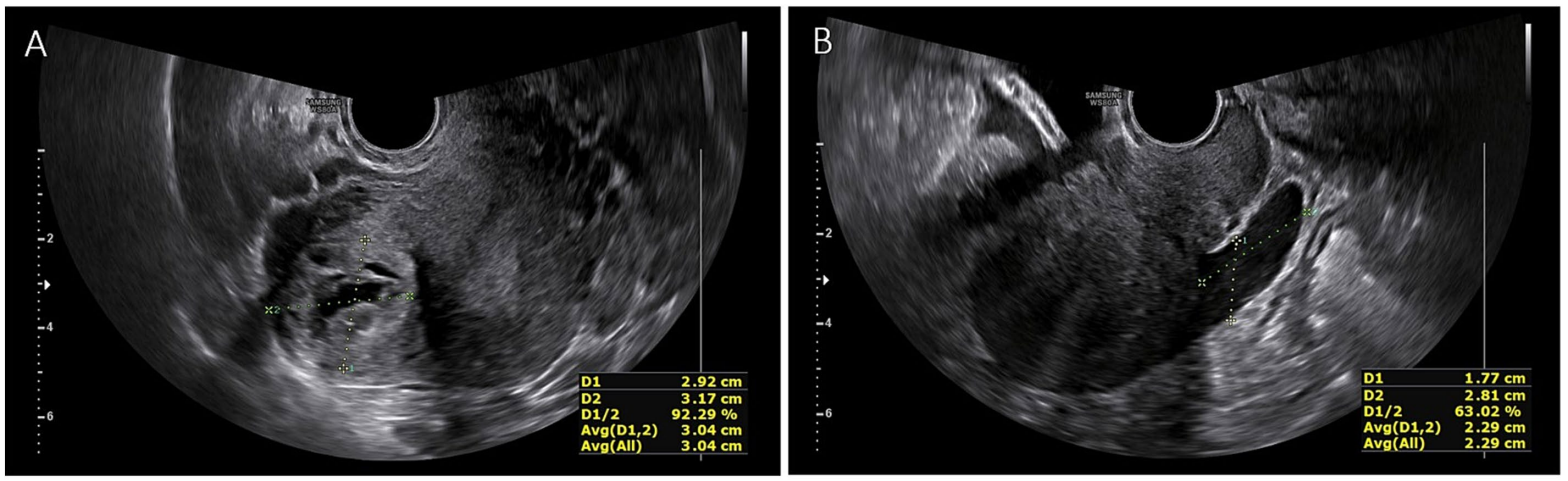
(A) Ultrasonographic finding. A 25×26 mm neoformation, with an intense peripheral vascularization in the right angular area suggesting an ectopic pregnancy; (B) Free fluid in the pouch of Douglas.
